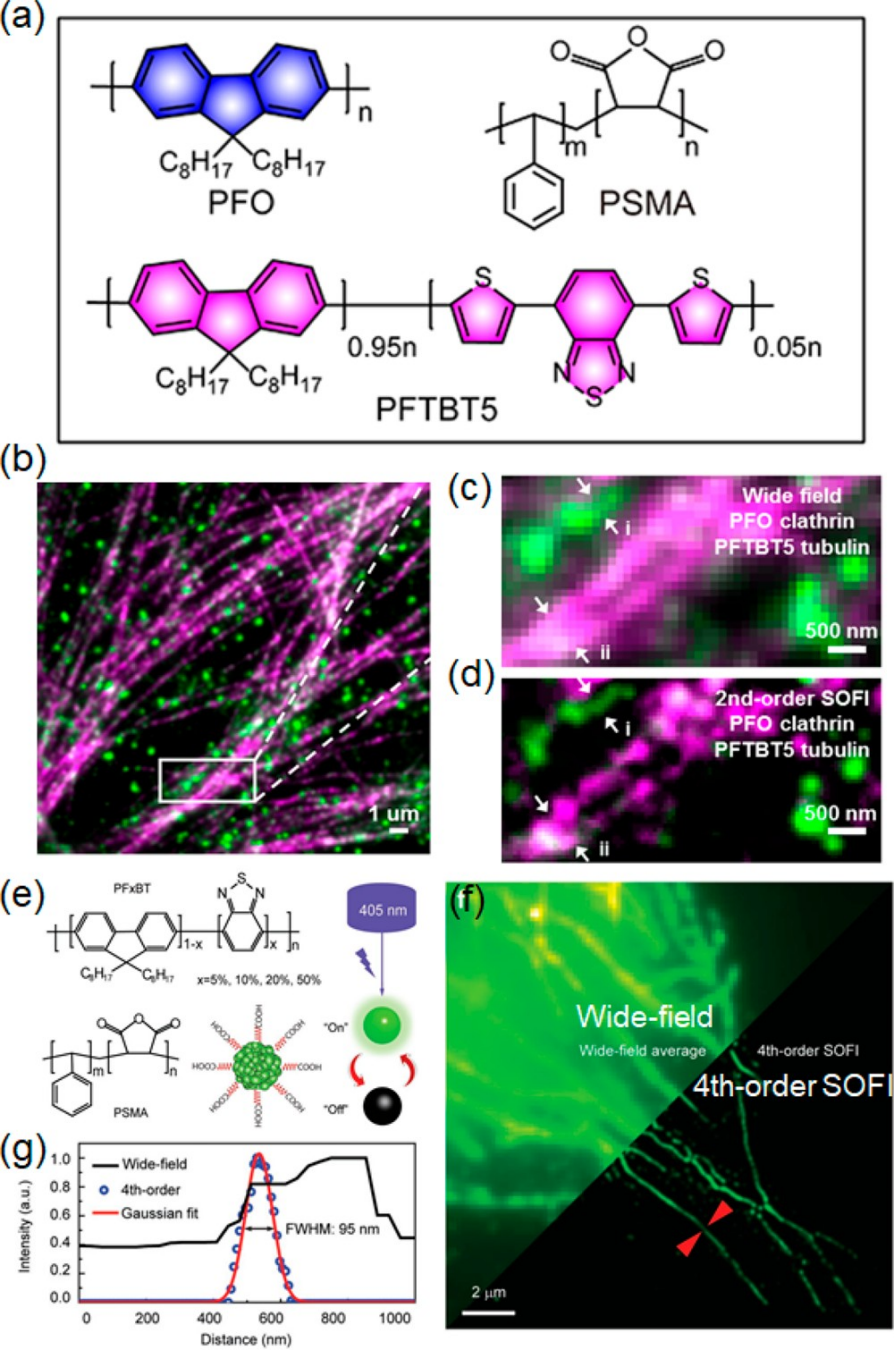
(a) Chemical structures of PFO, PSMA, and PFTBT5.
